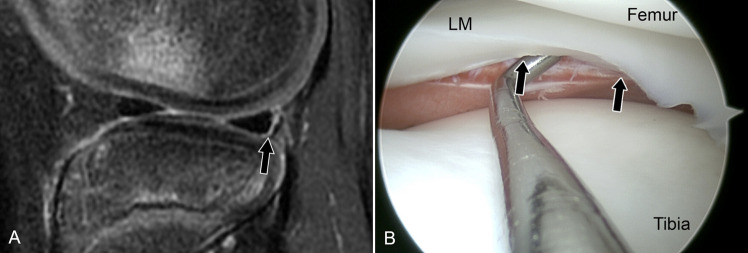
Meniscocapsular junction injury in a 12-year-old boy with an ACL tear. Sagittal proton-density-weighted fat-suppressed MRI (A) and arthroscopic view (B) show a longitudinal-vertical tear (arrows) of the posterior horn of the lateral meniscus (LM) near the meniscocapsular junction, with meniscal periphery contiguous with posterior meniscofemoral ligament. Arthroscopic view courtesy of Theodore J. Ganley, MD
